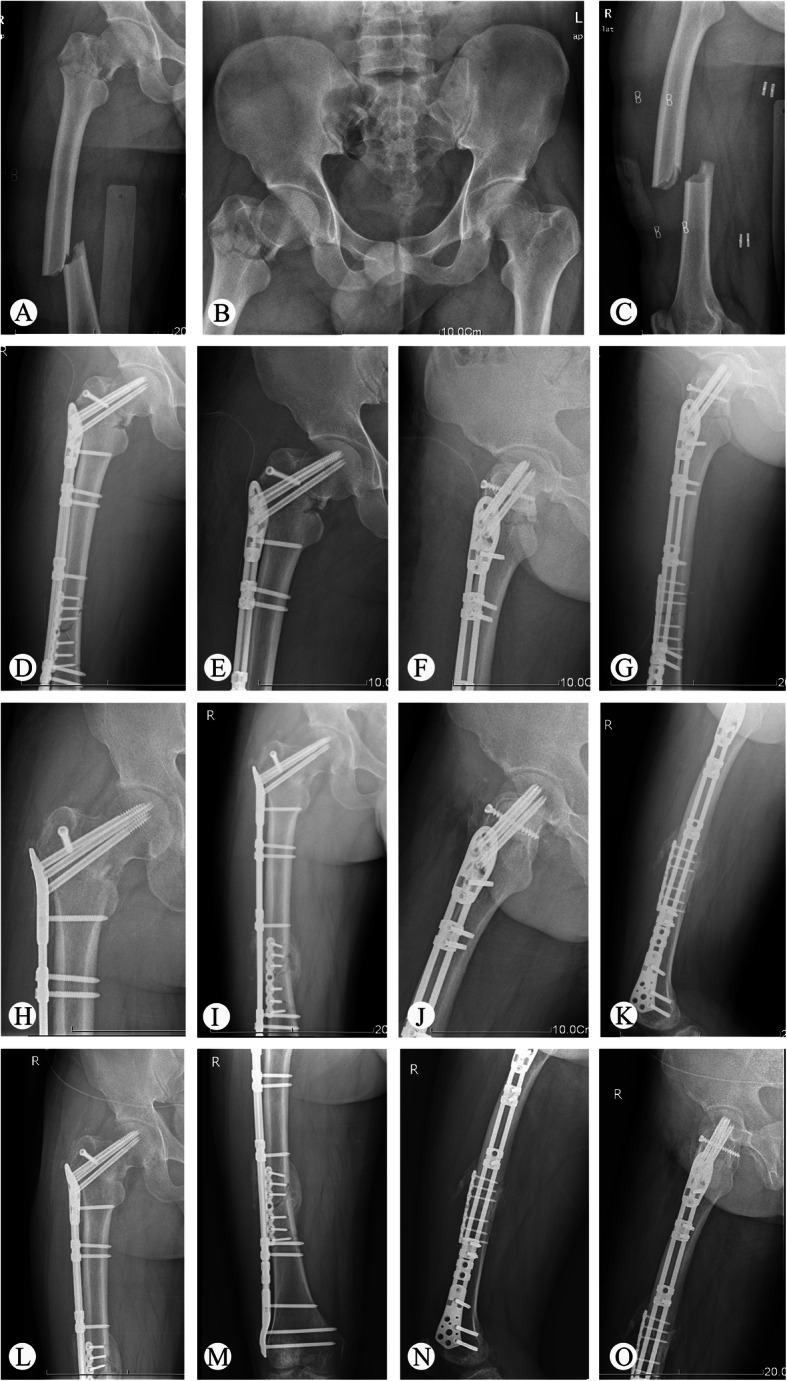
Representative images of patient 3. a–c X-ray at admission. d–g X-ray at 7 days after the operation. h–k X-ray at 4 months follow-up. l–o X-ray at last follow-up
